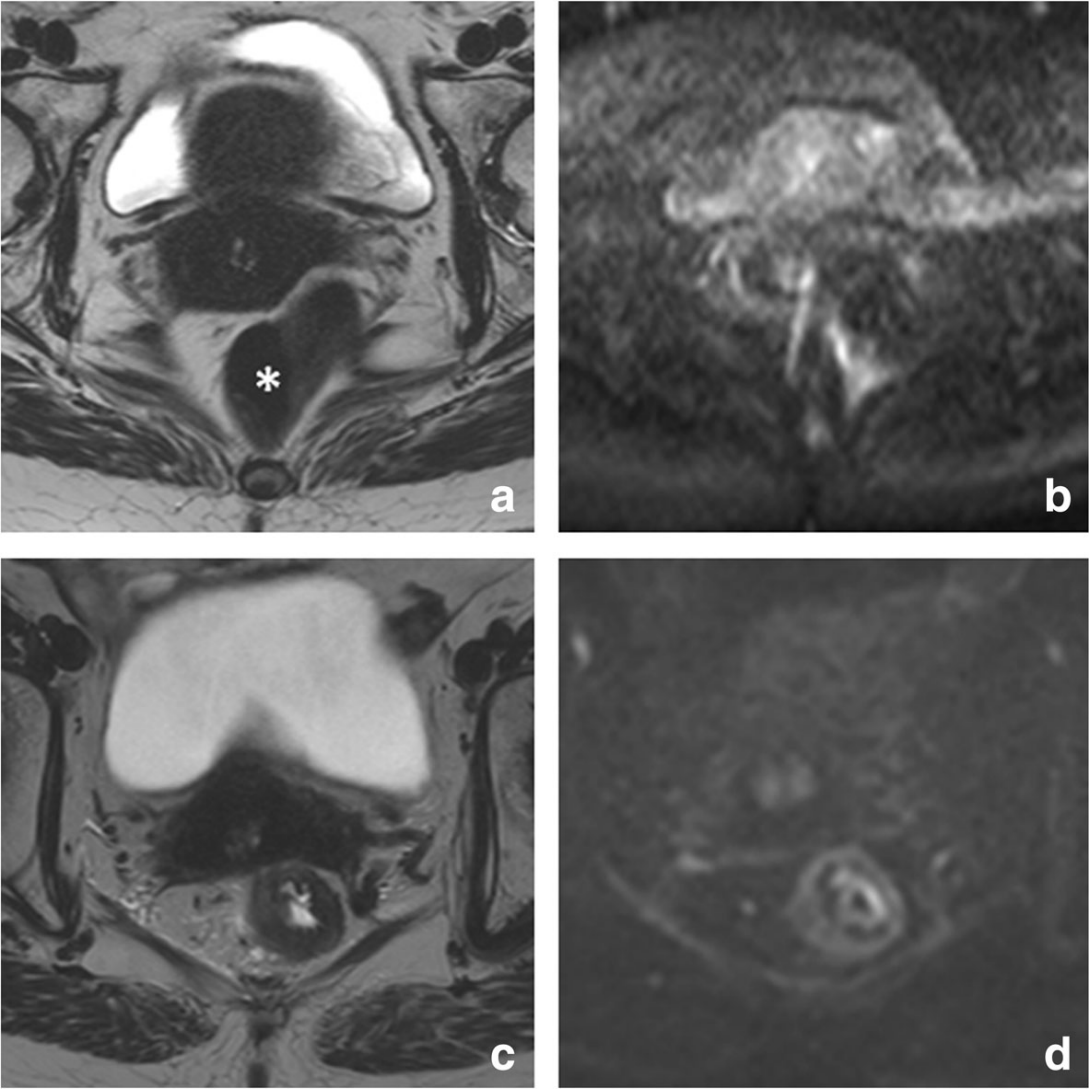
Effect of preparatory micro-enema. MR images of the same patient performed without (a T2-weighted; b b1000 diffusion-weighted) and with (c T2-weighted; d b1000 diffusion-weighted) a preparatory micro-enema. The patient is a clinical complete responder, followed with MRI as part of a watch and wait-program. Note the severe distortions on DWI (b) caused by gas in the rectal lumen (* in a). On the MRI performed after a preparatory micro-enema (self-administered by the patient 15 min before the MRI), there is no gas present in the rectum and the rectal wall is well discernible, both on T2-weighted and diffusion-weighted MRI
